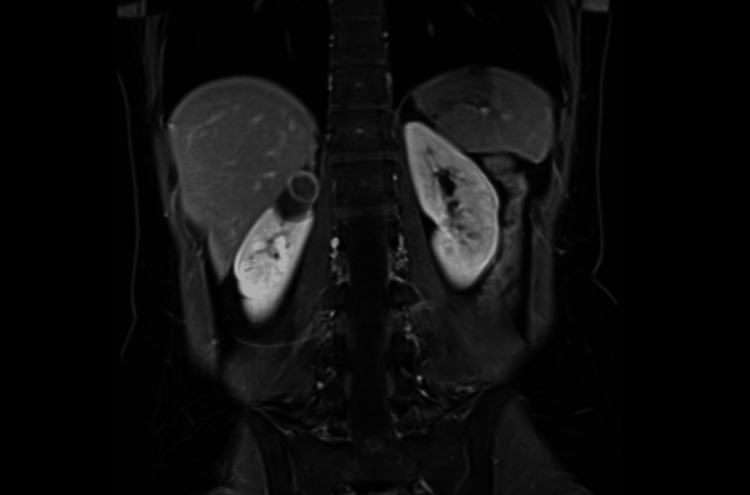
Coronal T1-weighted MRI showing a bilocular 35 x 24 mm lesion consistent with an involuting haemorrhagic cyst, classified as Bosniak IIF
